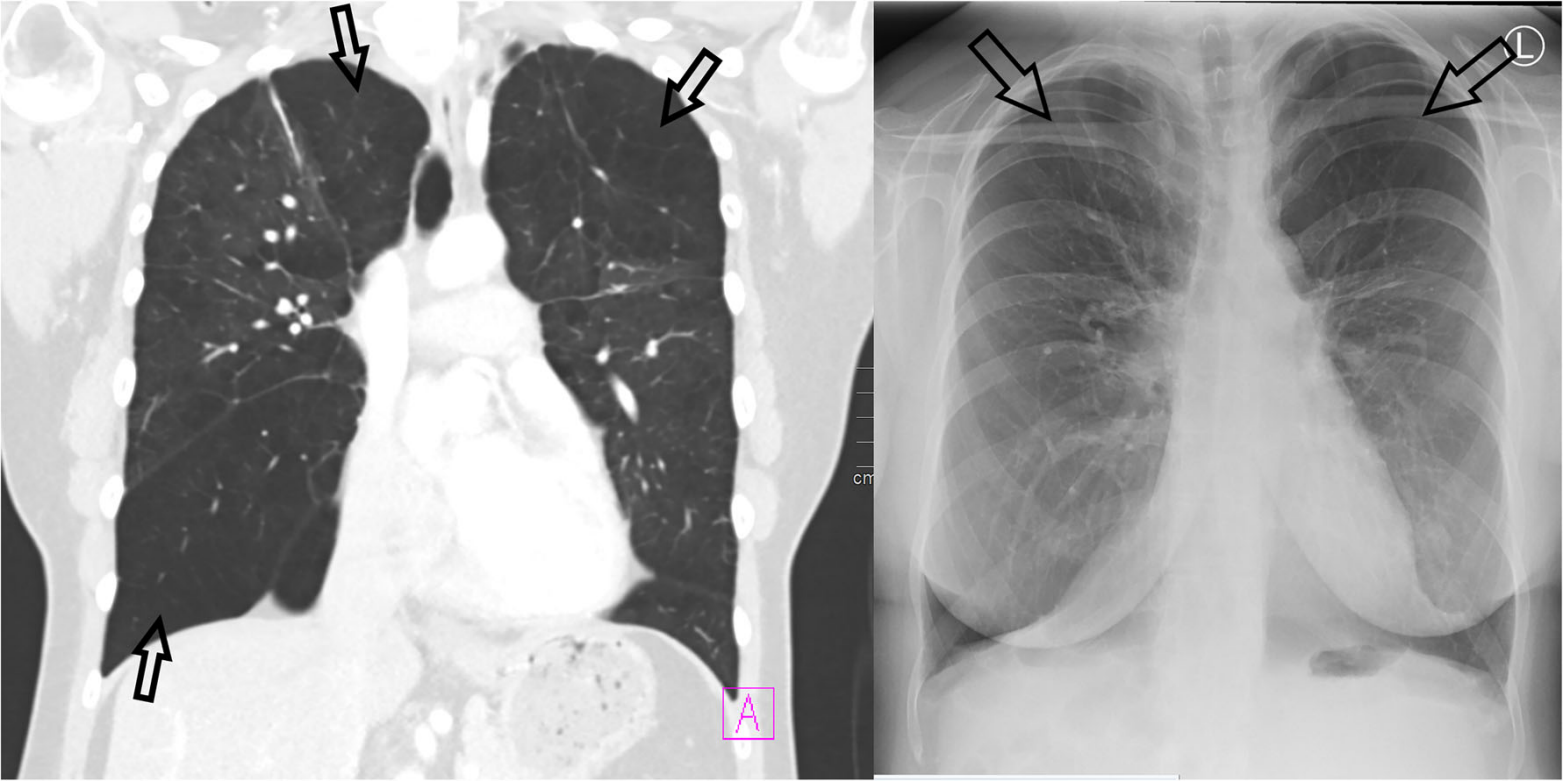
Contrast enhanced coronal chest CT (left) and X‐ray (right) images with marked panlobular emphysema in patient 2–1. There is abnormal hyperlucency of both lungs with paucity of the peripheral lung vessels (arrows)
